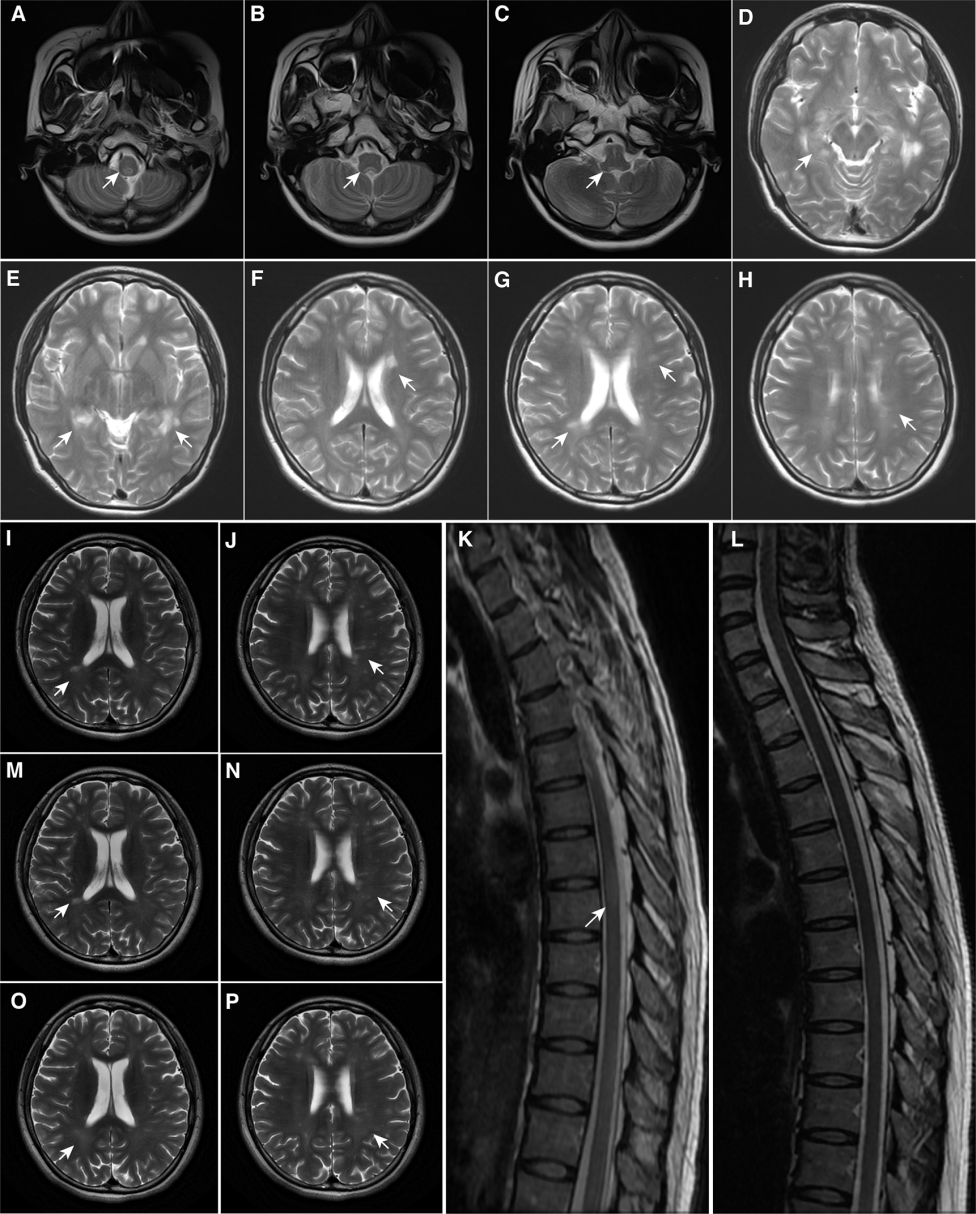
Magnetic Resonance Imaging Findings. (A–C) At first onset, MRI demonstrated patchy lesions in the medulla oblongata and dorsal pons; (D–F) At first recurrence, MRI demonstrated multiple lesions in the brain; (G, H) At second onset, MRI demonstrated pons lesions, consistent with the diagnosis of multiple sclerosis; (I–K) Before teriflunomide administration, MRI demonstrated new punctate enhancing lesions in the left frontal lobe and abnormal signals in the thoracic spinal cord at T6-7 of the skull and spinal cord; (L–N) After 6 months of teriflunomide use, MRI demonstrated that the lesions were reduced, especially in the spinal cord; (O, P) After 1 year of teriflunomide use, MRI demonstrated that the patient’s lesions were reduced. The arrowhead showing lesions in MRI. MRI, Magnetic resonance imaging.
